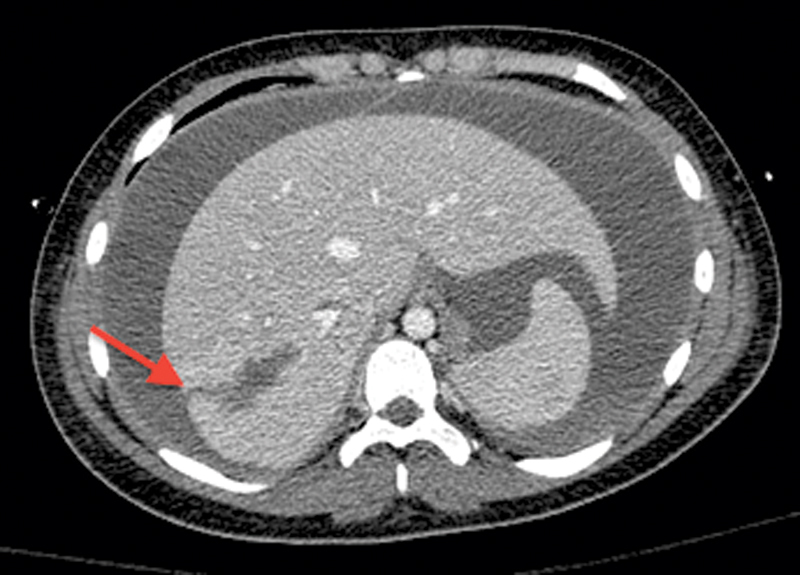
Case 2. CT (computed tomography) scan of the abdomen showing a large amount of free fluids in the abdomen.
